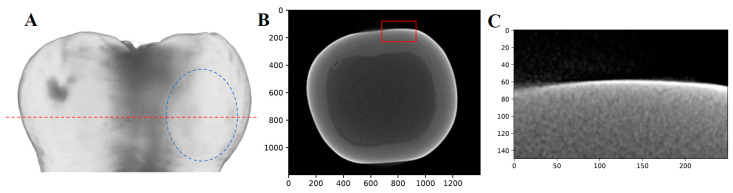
µ-CT results of sample M8 (8 days of demineralization): (A) Volume reconstruction with no visible lesion. The dashed blue oval indicates the region where the lesion is visible with the naked eye, the dashed red line indicates the plane where the transverse view B is taken. (B) Transverse view of the sample with the red box indicating where the lesion should be visible. (C) Enlarged view of the red box seen in B, with no lesion visible.
